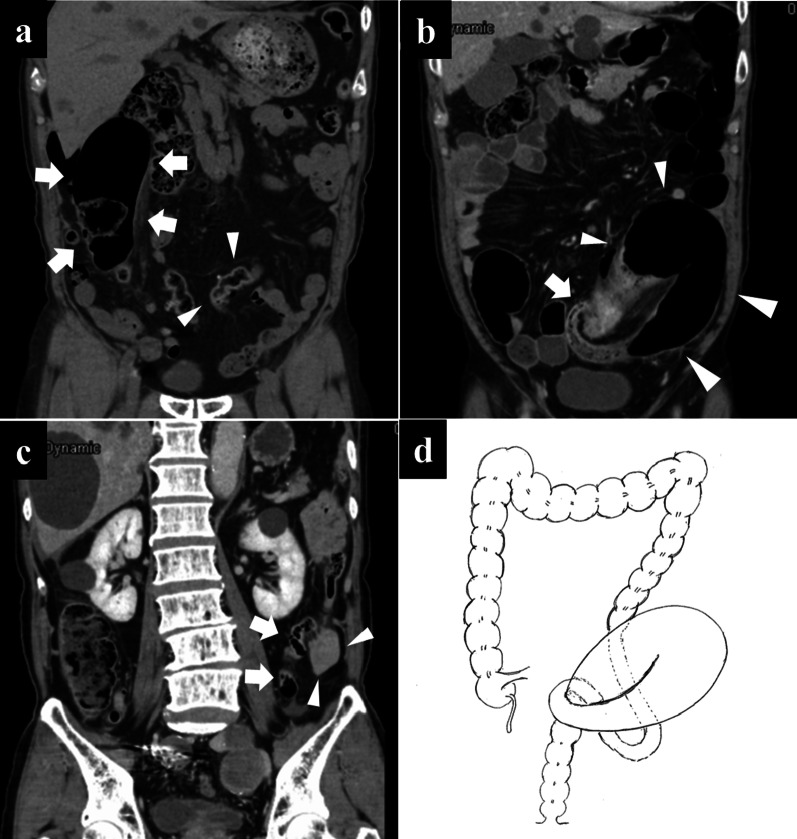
First abdominal CT shows dilated sigmoid colon on the right side (arrows) and shifted descending colon towards the medial side (arrowheads) (a). Second abdominal CT shows strangulation (arrows) and the oral dilated sigmoid colon (arrowheads) (b). Second abdominal CT also shows descending colon shifted to median side (arrows) and small intestine located at the lateral side of the descending colon (arrowheads) (c). The patient presented sigmoid volvulus with PDM (d)
